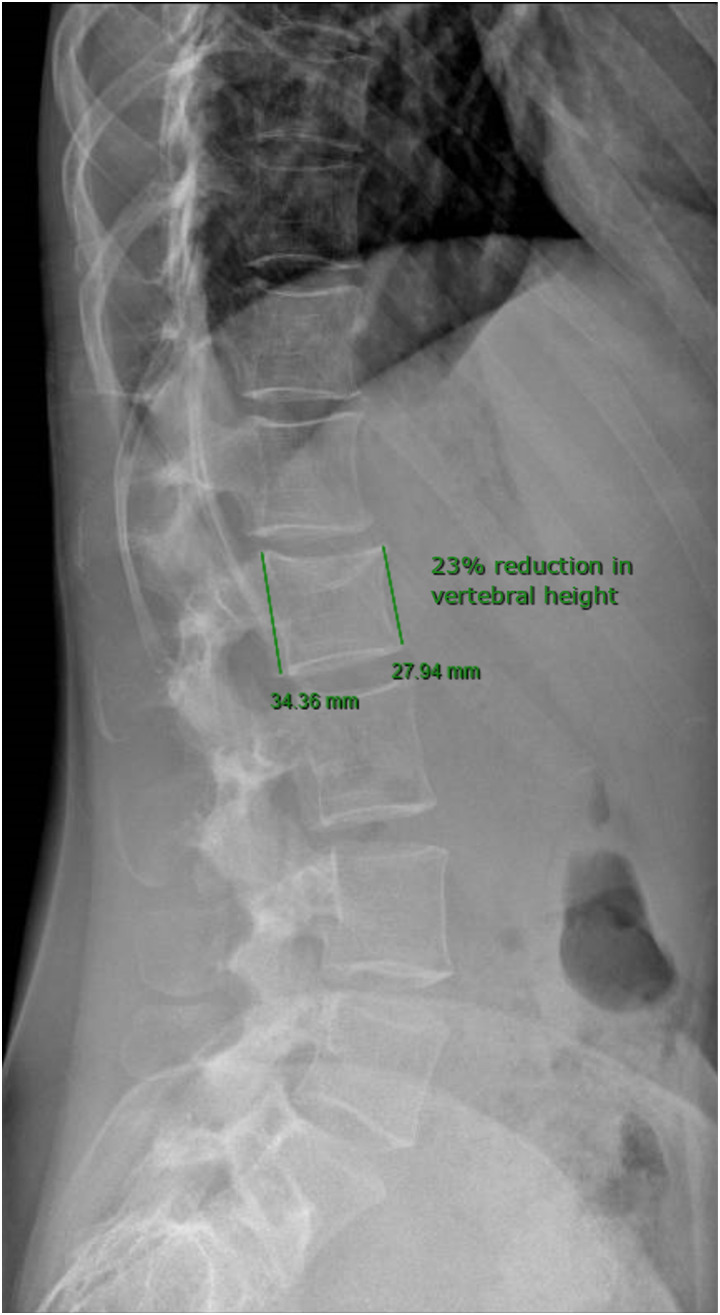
Thoracic and lumbar spine lateral view X-ray showing a mild fracture of L2 vertebral body without other vertebral deformities.
